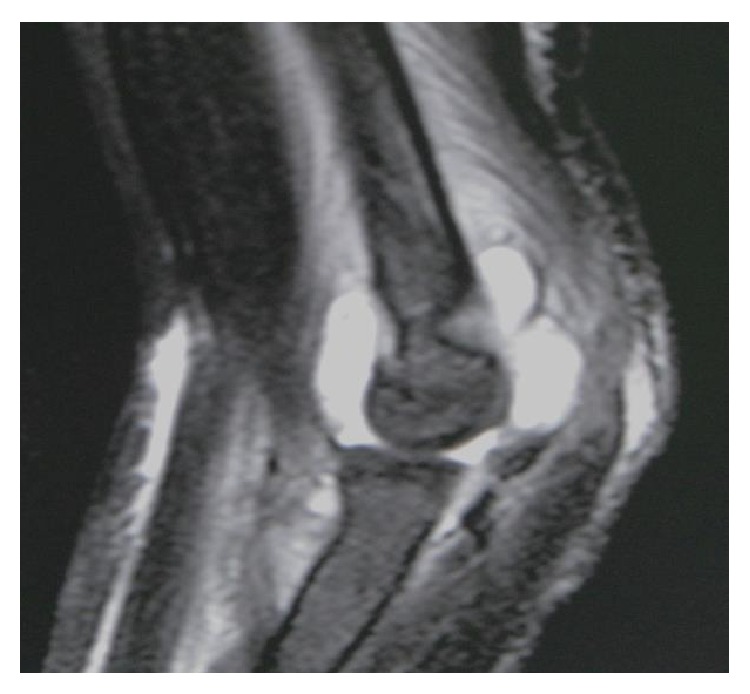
A fat suppressed T2-weighted MRI. A sagittal MRI revealed a presence of joint fluid pooling in the elbow and swelling of soft tissues around the joint.
